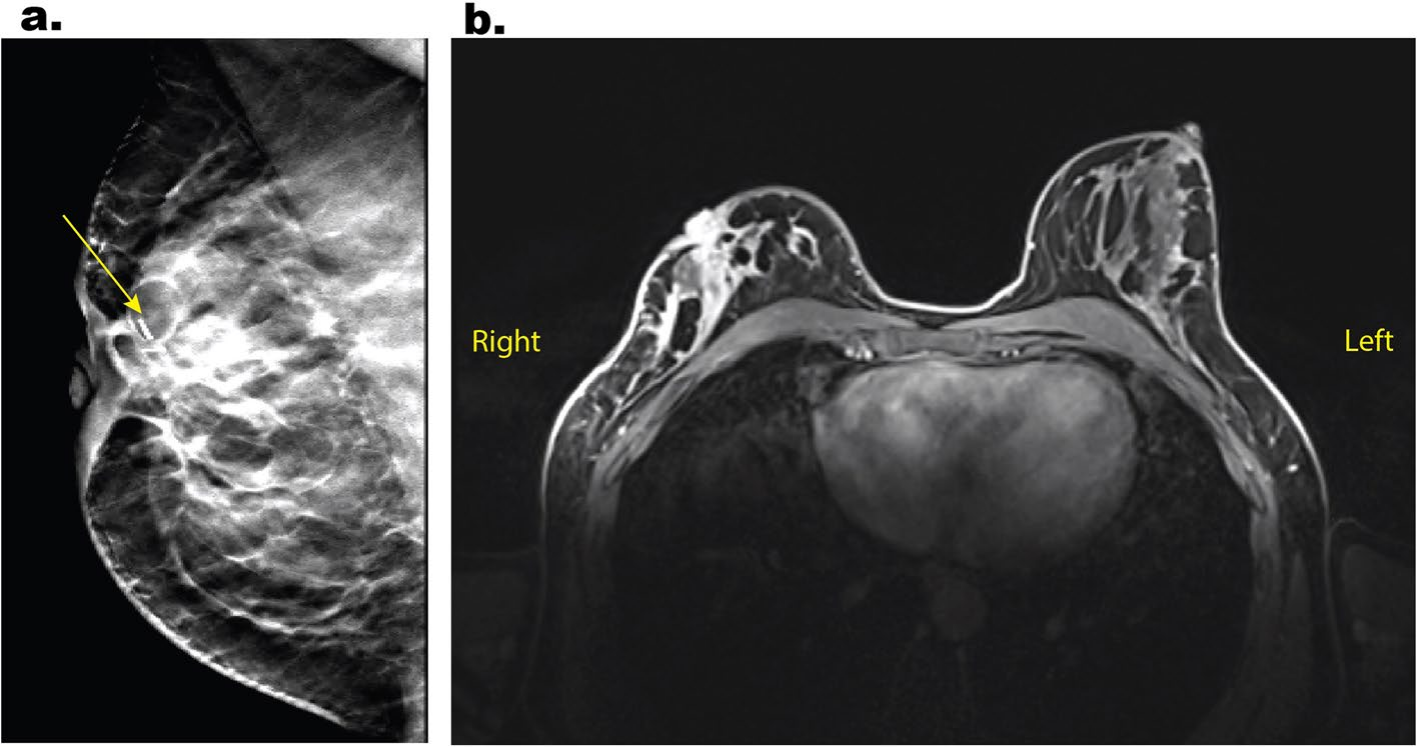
Mammogram and magnetic resonance imaging (MRI) showing ipsilateral breast cancer relapse before neoadjuvant antihormone therapy. a Mammogram of the right breast in a mediolateral oblique view with suspicion of a relapse BIRADS 4, ACRb. It shows increased parenchymal density of the upper right quadrant compared to the previous follow-ups, progressive microcalcifications medial to the clips (arrow), and progressive mamilla retraction. b T1-weighted transverse axial dynamic contrast-enhanced MRI with fat suppression showing asymmetric accumulation of contrast and restricted diffusion of the whole right breast including the mammilla. There is subcutaneous edema of the right breast, but no sign of lymph node metastases or distant metastases
